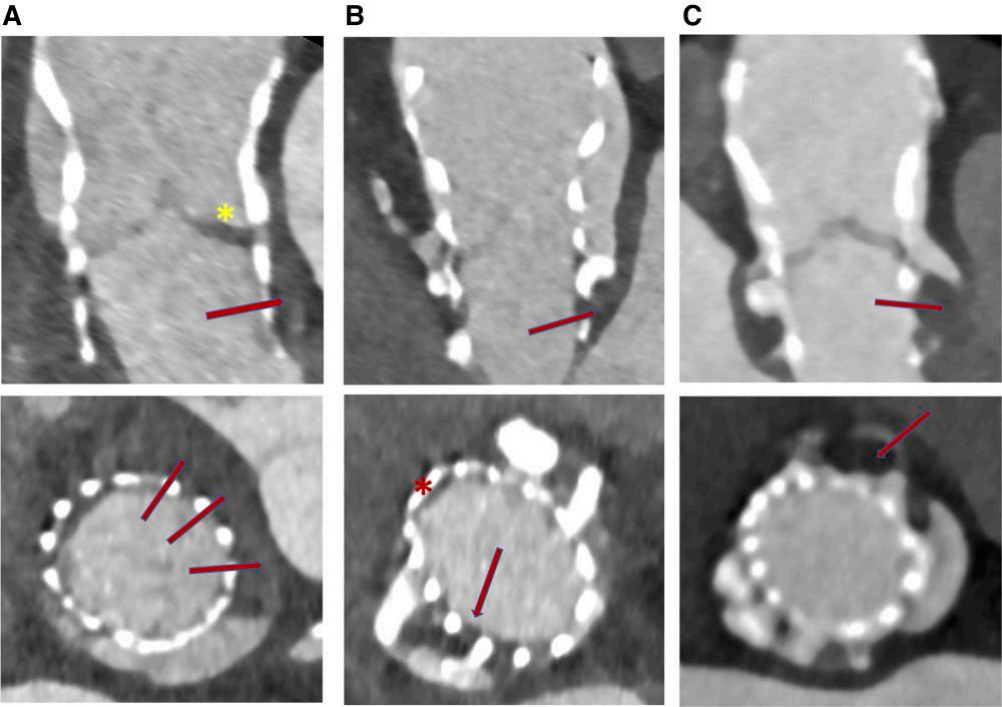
(*A-C*) Anatomic sinus thrombosis visualised with multi-detector computed tomography in long and short axes (red arrows). Asterisk in panel (*A)* indicates association with leaflet thrombosis. Asterisk in panel (*B*) indicates high eccentricity index.
